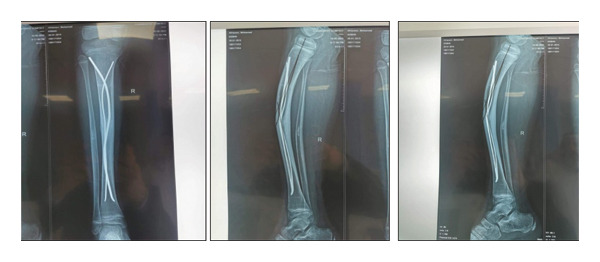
Postoperative radiograph demonstrating an elastic stable intramedullary nail (ESIN) in the right tibia following surgical fixation for the midshaft fracture with anterior angulation.
